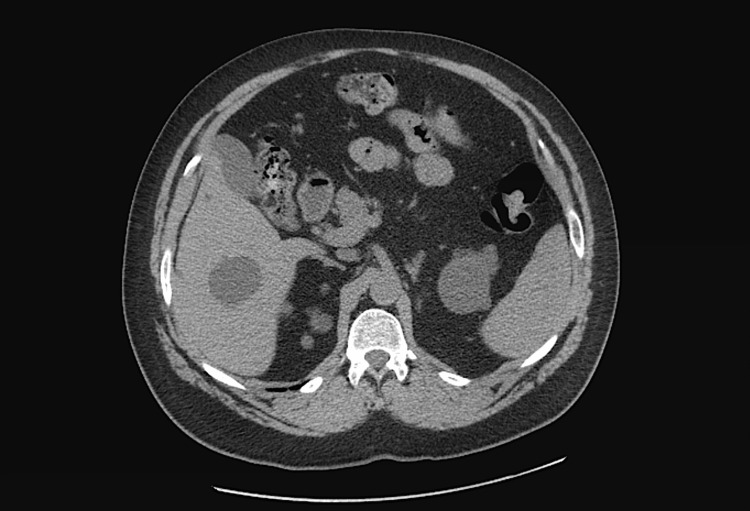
Abdominal CT scan showing hepatic cyst
